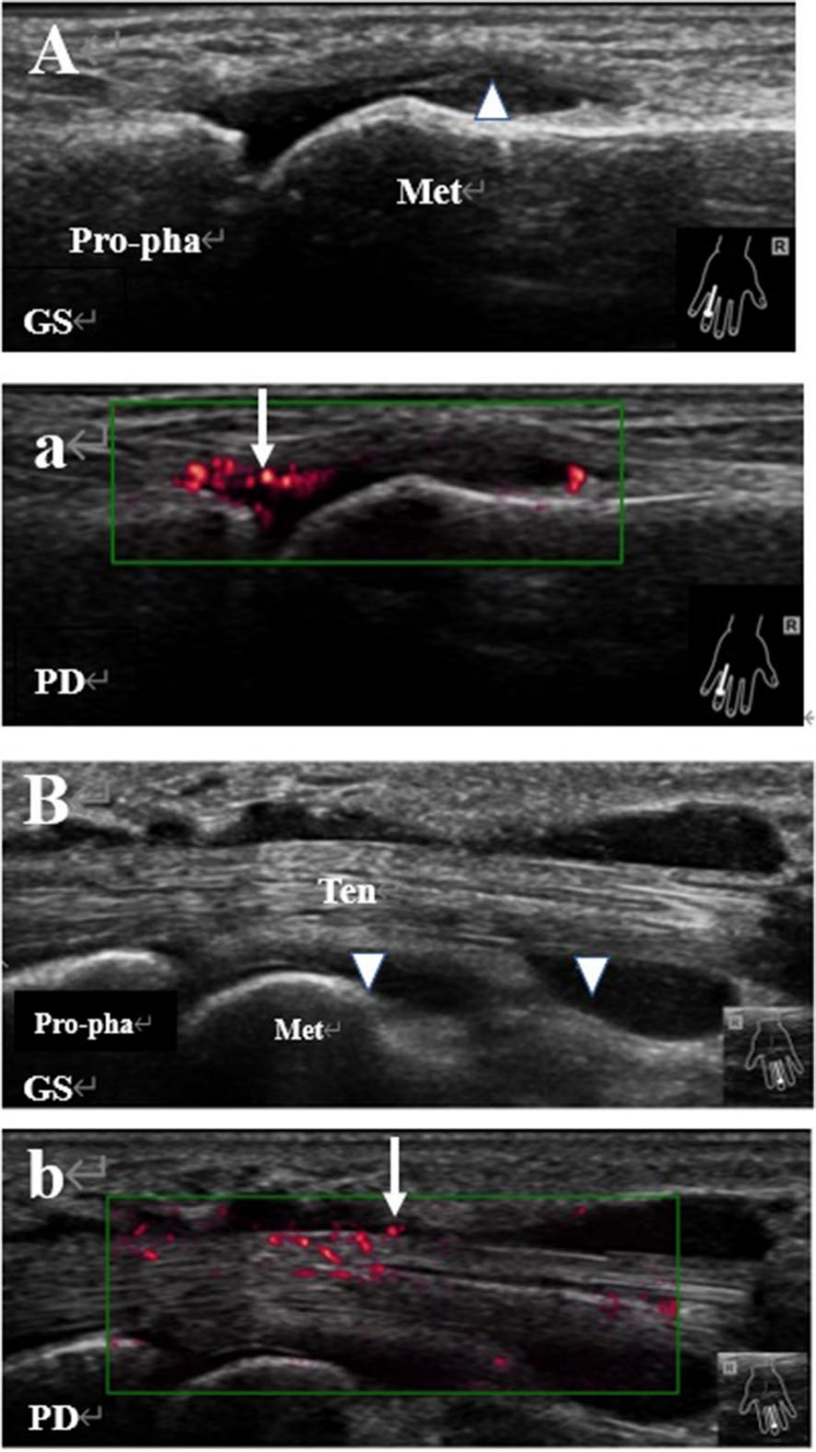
Representative images of synovitis and tenosynovitis in a patient with SNRA. (A) Synovitis in the fourth metacarpophalangeal joint of the right hand, with the GS grade of 3 (triangle). a. Blood flow signals in the thickened synovium upon PD (arrow), with the PD grade of 2; (B) Tenosynovitis in the flexor tendon of the third digit of the right hand, with the GS grade of tendon synovial sheath of 3 (triangle); b. Blood flow signals observed near the tendon synovial sheath upon PD (arrow), with the PD grade of 2. Abbreviations: GS Grayscale, PD power Doppler, Met metacarpal, Pro-pha proximal phalanx, Ten flexor tendon.
